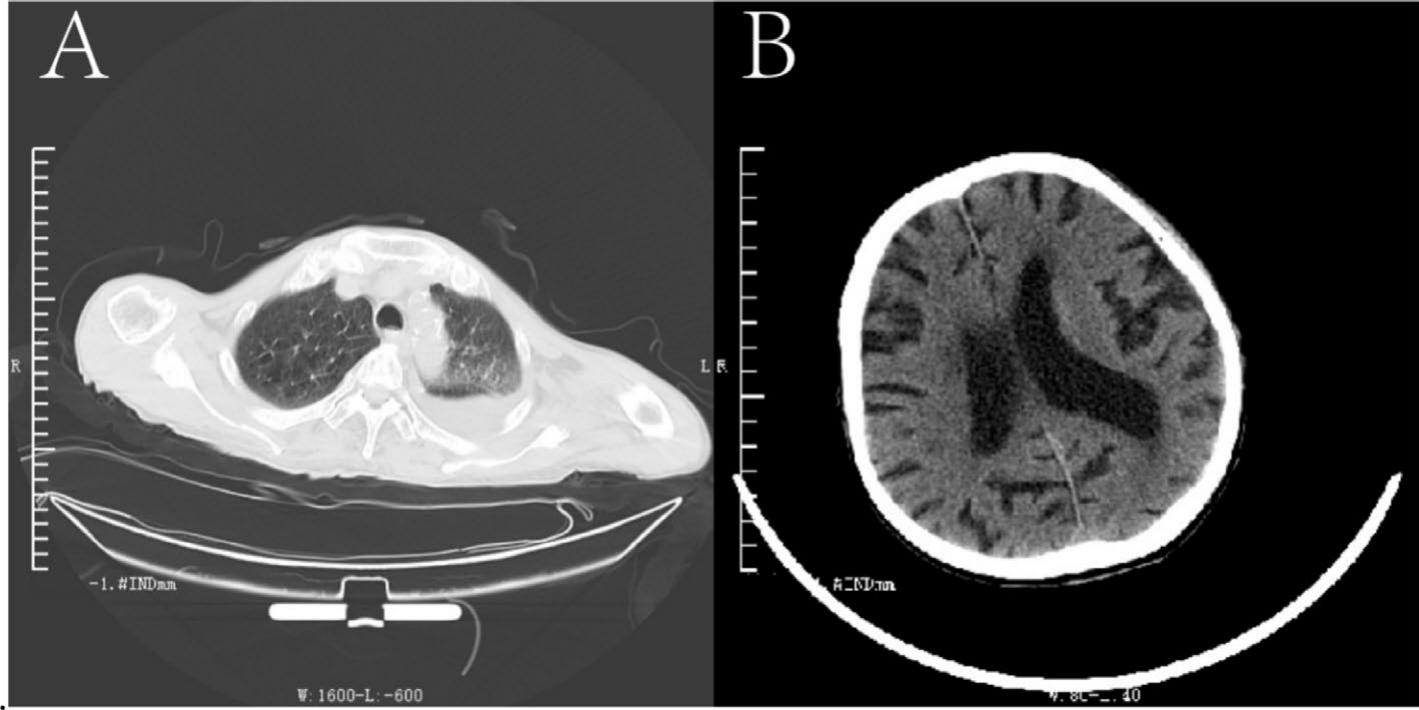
Pneumonia and cerebral infarction on September 19, 2017: (A) The chest CT plain scan shows aspiration pneumonia in both lungs, with a small to moderate amount of pleural effusion in the left lung. (B) The head CT plain scan indicated cerebral infarction in the right temporal lobe in September 2017.
